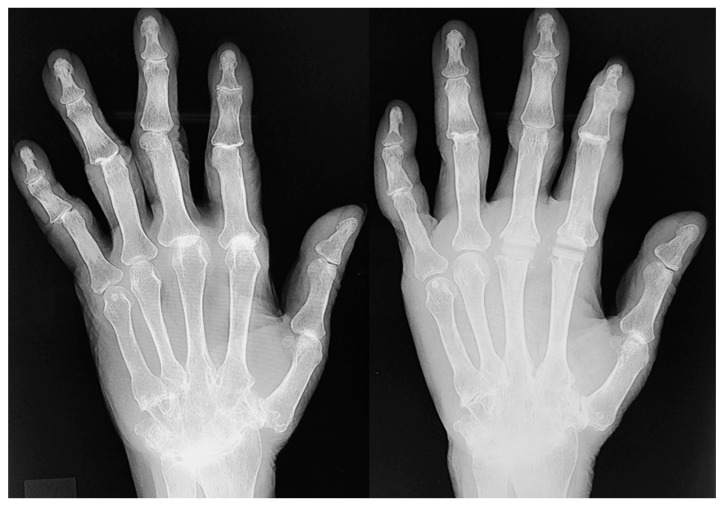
Radiographs of “hybrid finger arthroplasty” ((left): preoperative; (right): postoperative). The MCP joints of the index and middle fingers were replaced with implants, and the ring and little fingers underwent joint-preserving arthroplasty.
